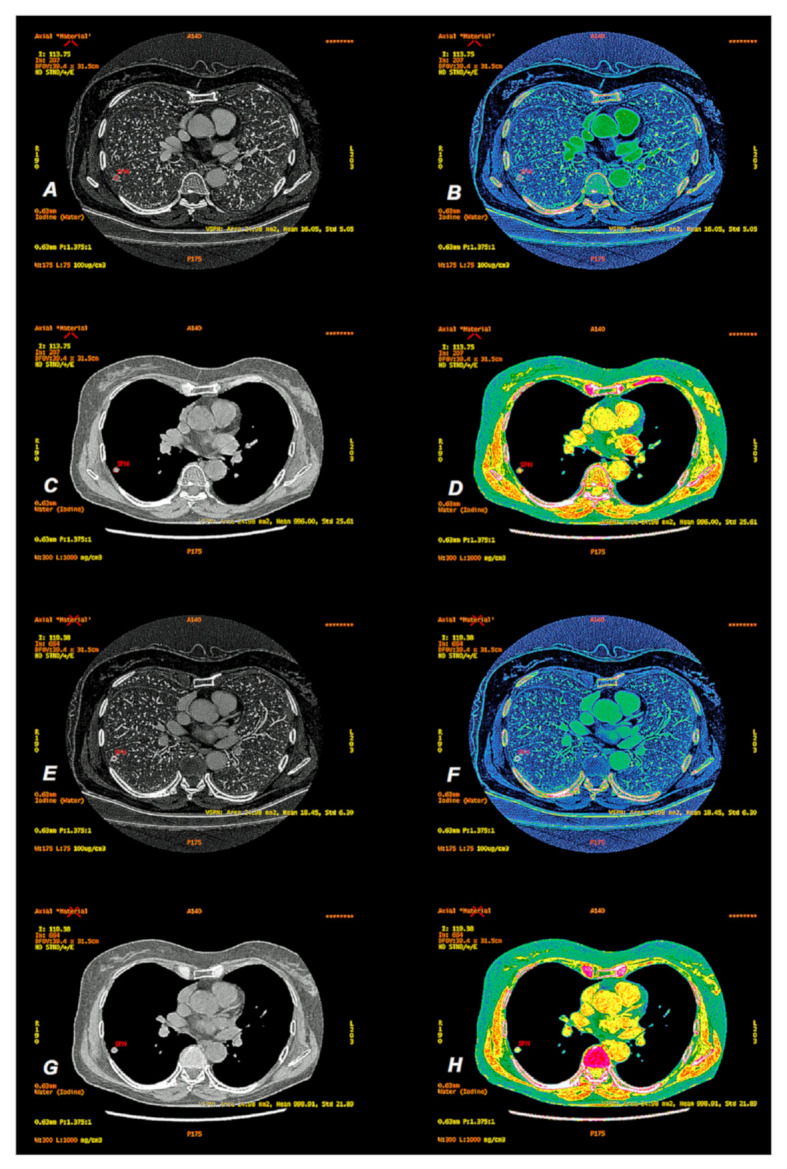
Peripherally located tumor of the right lung (SPN). (A,E) show images depending on the concentration of iodine (IC) in the arterial (AP) and venous (VP) phases of the contrast enhancement in the linear gray option and their modification on the Colormap French map to improve the contrast of details (B,F). The iodine concentration in the lesion is 16.05 ± 5.05 (×100 µg/cm^3^) in AP and 18.45 ± 6.30 (×100 µg/cm^3^) in VP. Water concentration-dependent (WC) images are the result of contrast medium subtraction, and as virtual native images (VNC), they can replace native scanning in conventional CT. (C,G) show examples of iodine subtraction in AP and VP on standard Colormap Linear gray and French modification (D,H). The SPN measurement values were 996 ± 25.61 mg/cm^3^ (AP) and 998.91 ± 21.89 mg/cm^3^ (VP).
